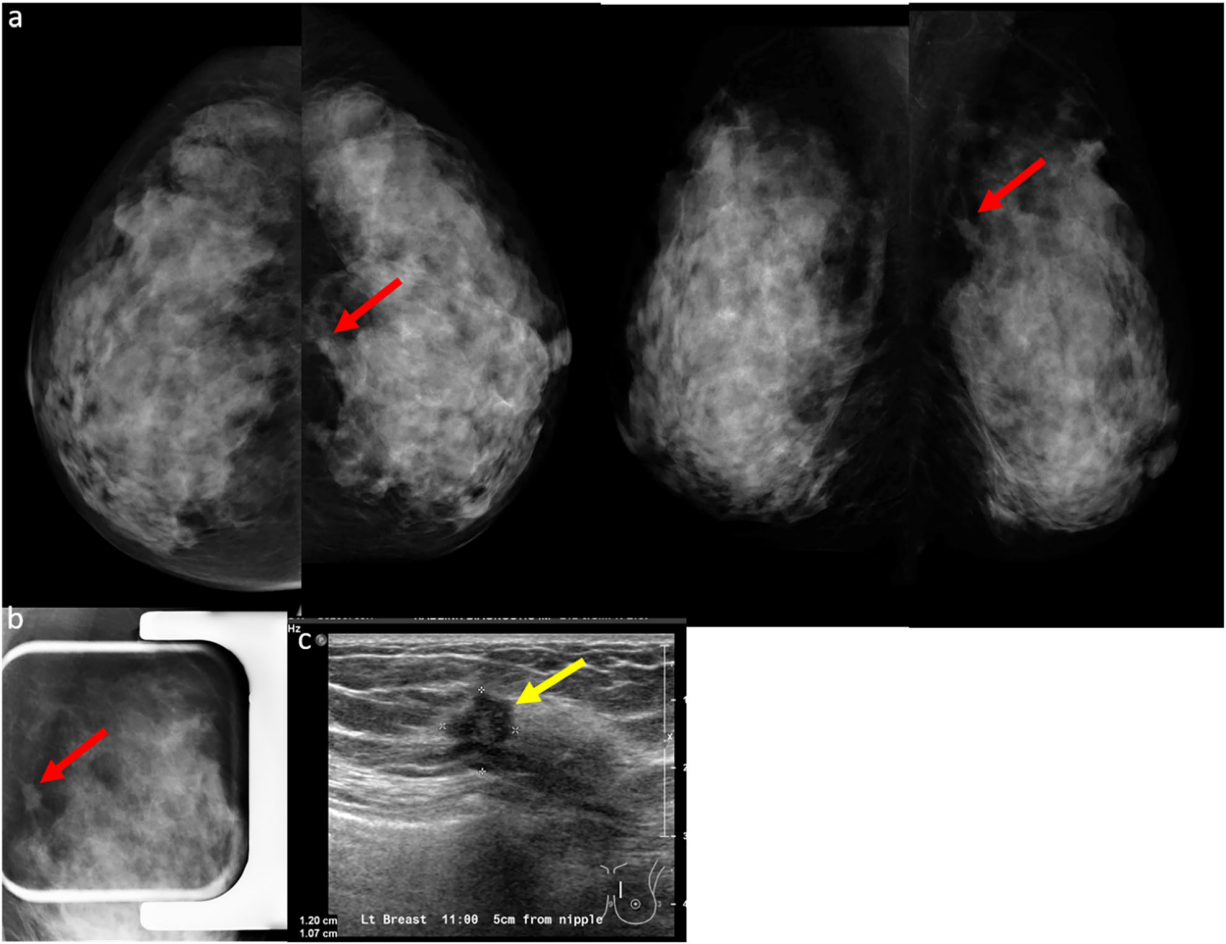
a A 45-year-old woman underwent a baseline screening mammogram, which was interpreted as normal by one reader. A second reader identified a possible isodense mass in the upper inner quadrant of the left breast, projected over the retromammary fat (red arrows, left CC and MLO mammograms). b Spot compression left lateral-medial mammogram demonstrated an irregular mass with spiculated margins (red arrow) in the area of concern. c Targeted ultrasound demonstrated a correlating irregular hypoechoic mass with indistinct margins (yellow arrow). Ultrasound-guided core biopsy revealed invasive ductal carcinoma
